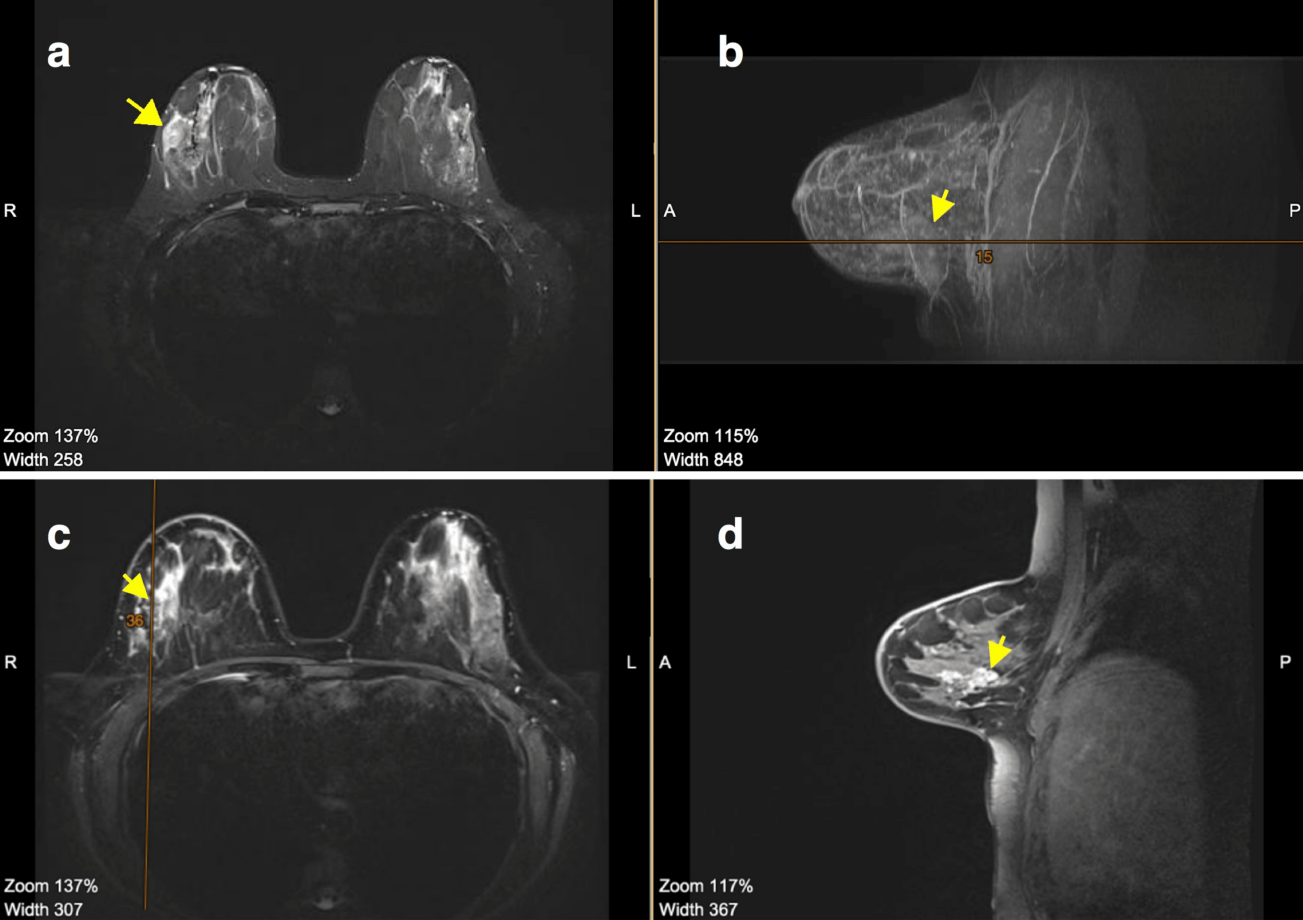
Comparison between baseline MRI and post-neoadjuvant MRI of the breasts showing partial regression of the lesion (a) Baseline MRI of the breast - axial section with an arrow pointing to the lesion. (b) Baseline MRI of the right breast - sagittal section with an arrow pointing to the lesion. (c) Post-neoadjuvant MRI of the breast - axial section with an arrow pointing to the lesion. (d) Post-neoadjuvant MRI of the right breast - sagittal section with an arrow pointing to the lesion. R: Right; L: Left; A: Anterior; P: Posterior; MRI: Magnetic resonance imaging
